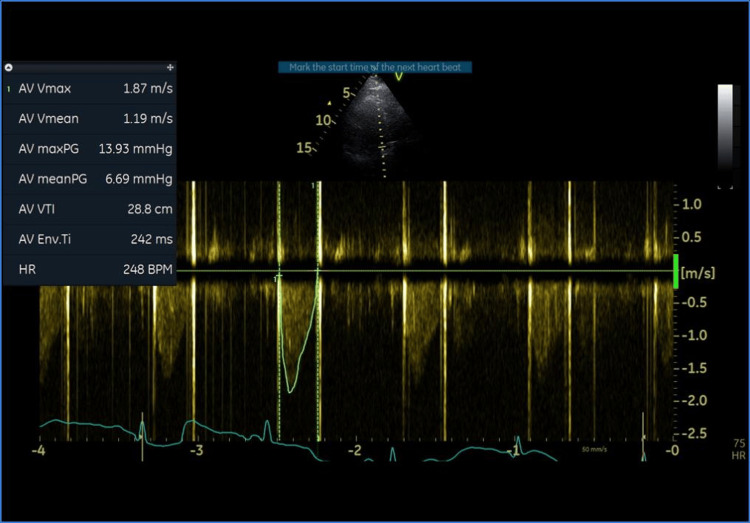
Post-operative transthoracic echocardiogram of aortic valve prosthesis with a mean gradient of 6 mmHg.
